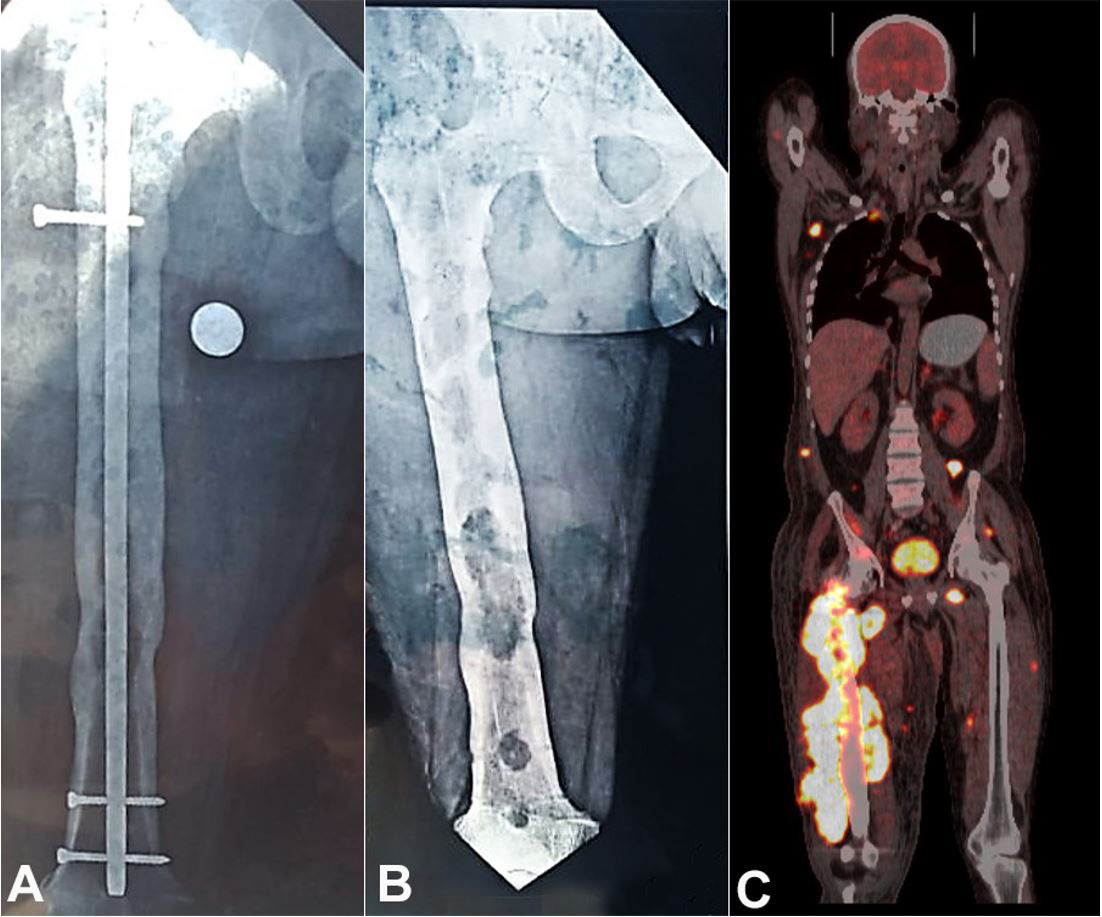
A and B – Antero-posterior and lateral plain radiographic view of the right lower extremity showing a femur with and without metallic rod and fixation screws. The ill-defined, expansile, intramedullary lytic lesions are seen involving metaphyseal and diaphyseal regions. C – Coronal fused PET/CT showing increased FDG uptake as the large skeletal lesion involving right femur and few enlarged lymph nodes along with subcutaneous and intramuscular deposits.
